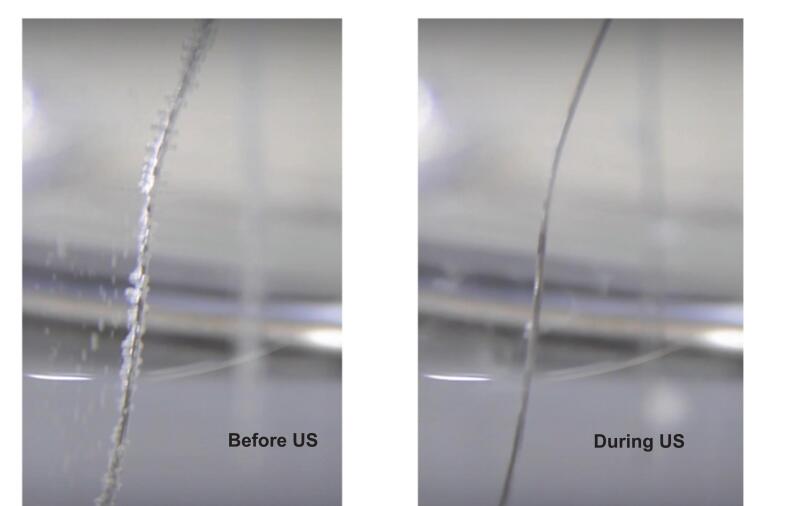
Hydrogen gas flux on Pt wire before and during ultrasonication (US) [23].
